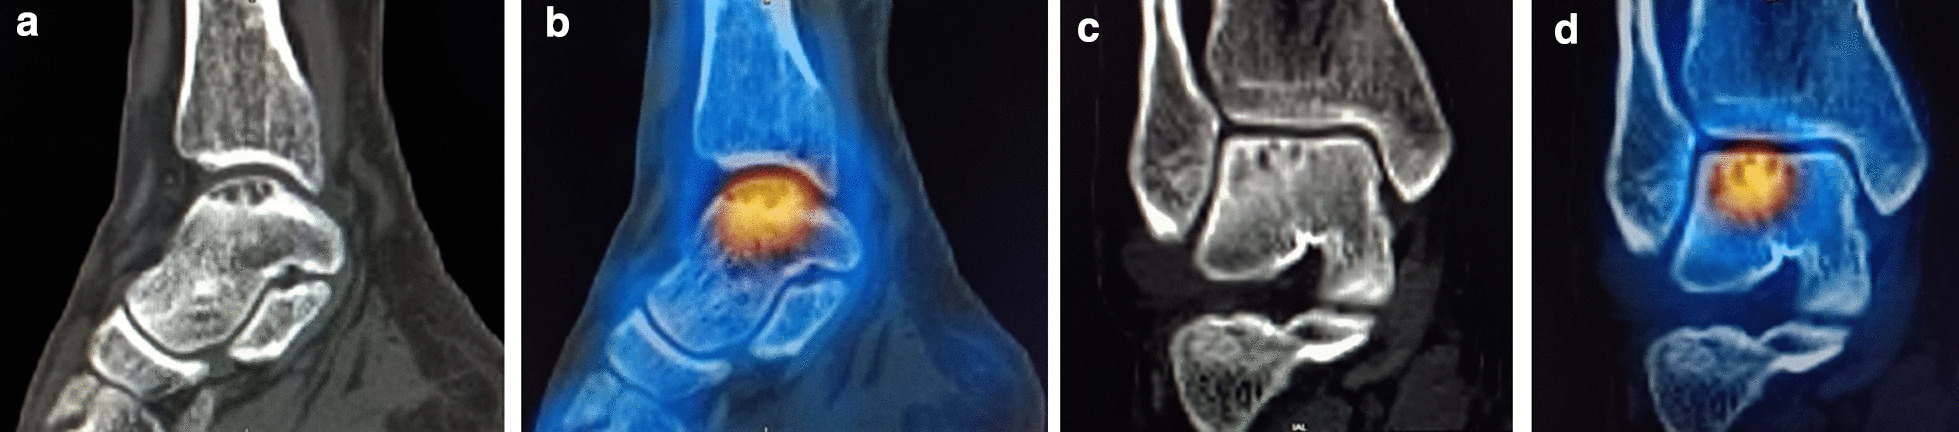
A 57-year-old man with left ankle pain for more than 5 years, history of a minor sprain, and other medical histories. CT sagittal (a) and coronal (c) images show multiple consecutive small bursae with sclerosis of the surrounding bone visible in the subchondral bone of the talar fornix. This is consistent with a type I cystic lesion. SPECT/CT fusion images (b,d) show that the area of tracer concentration in the talar fornix corresponds to the cystic area and the surrounding sclerotic bone
